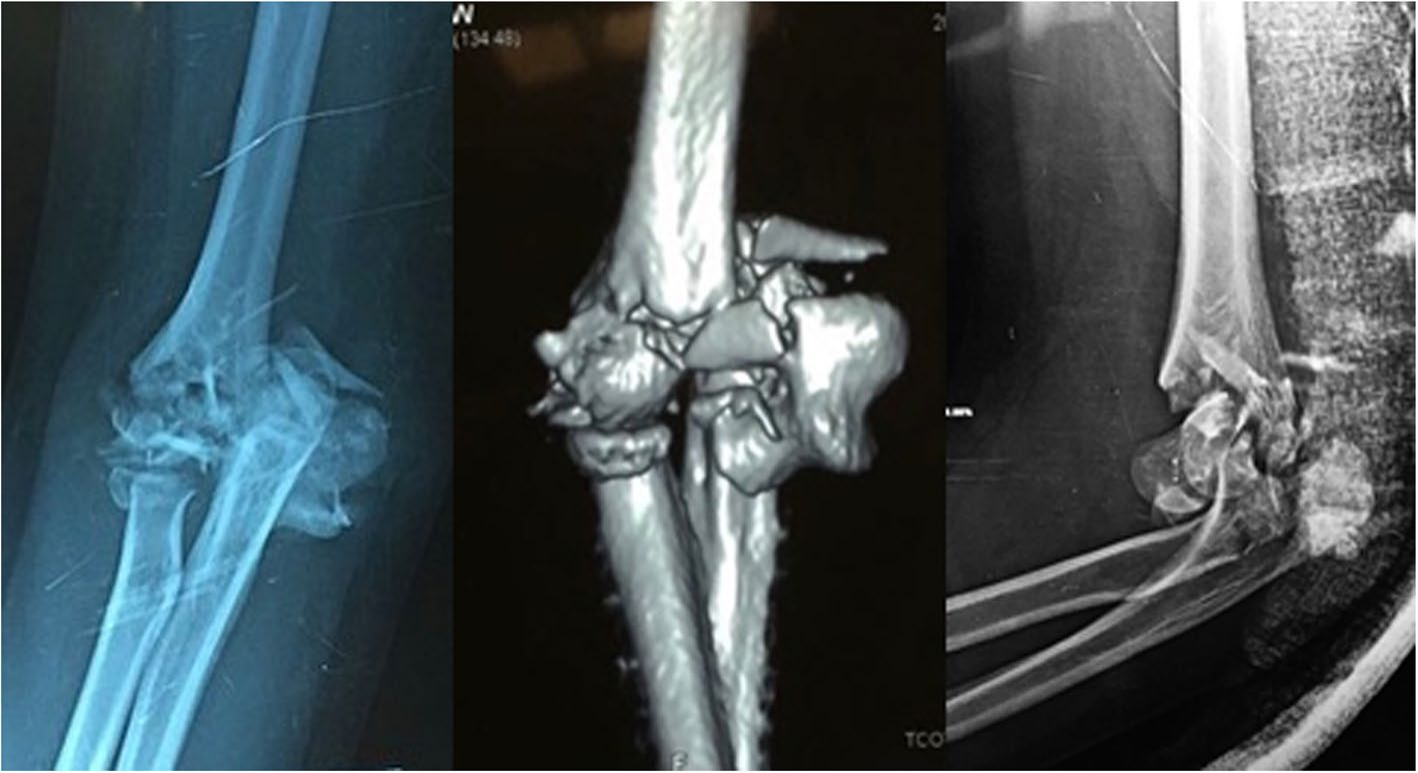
X-rays at presentation of a 19 years’ old female (case #14) with highly comminuted fracture of the distal humerus at the dominant hand
